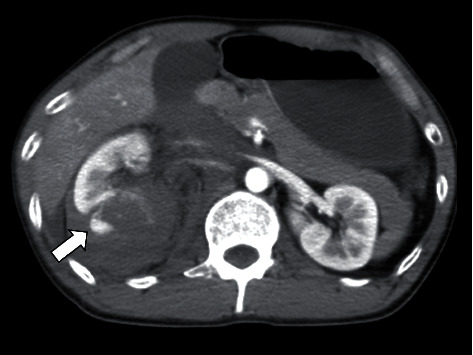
Enhanced abdominal computed tomography (CT) on arrival. CT showed extravasation of contrast medium from the injured right kidney. An accompanying massive retroperitoneal hematoma had pushed up the visceral organs into the ventral side.
